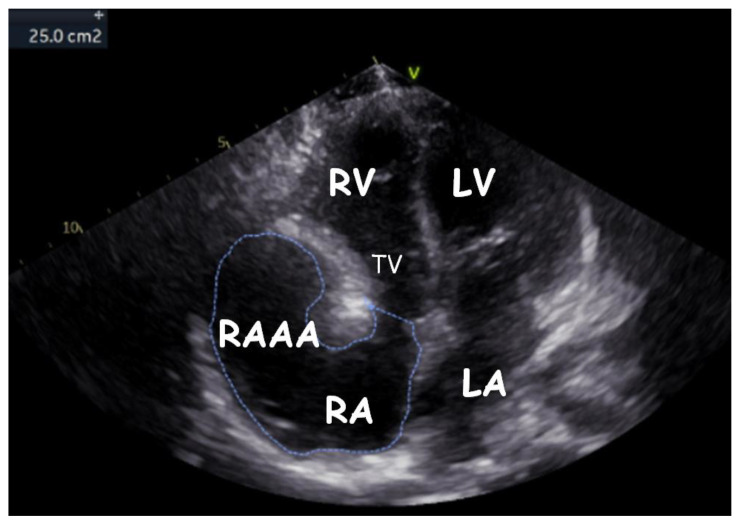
Transthoracic echocardiogram ten years later. Modified subcostal view showing the gigantic RAAA and the RA (estimated surface or RAAA + RA = 25 cm^2^).
